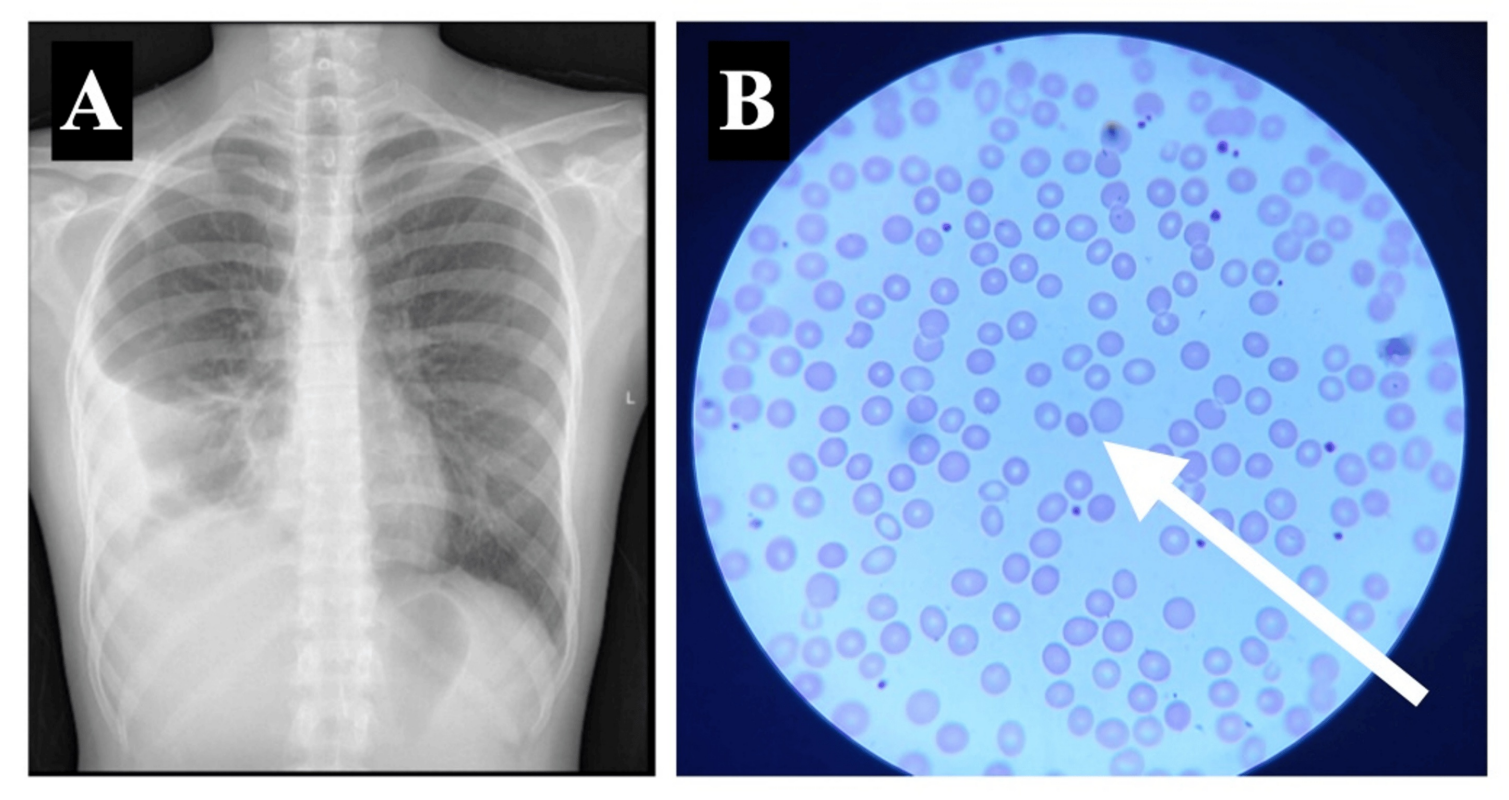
Chest radiograph and peripheral blood smear findings during discharge. (A) Chest radiograph obtained during discharge showing partial resolution of infiltrates. (B) Peripheral blood smear obtained on discharge showing microspherocyte (white arrow) and reduced agglutination of RBC. RBC: red blood cell
